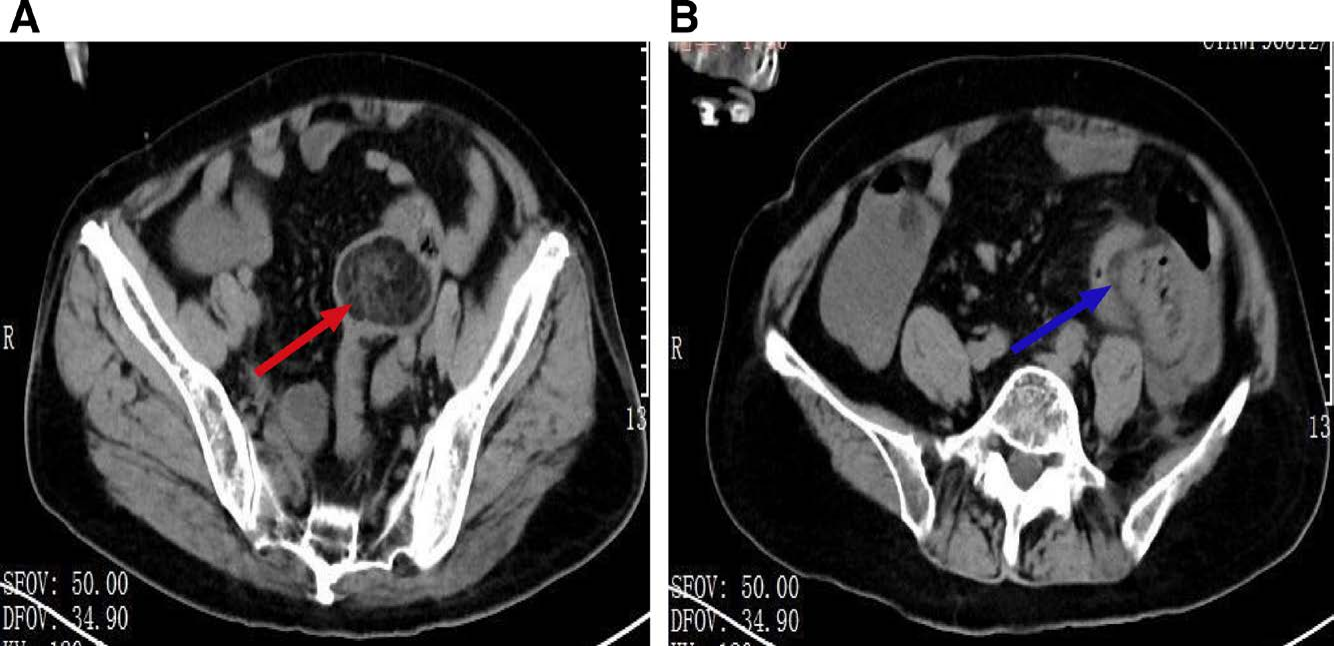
CT examination results. (A) Axial abdominal CT image demonstrates a heterogeneous mass with fatty density within the sigmoid colon (red arrow) and (B) axial abdominal CT image shows intussusception within the abdominal cavity (blue arrow). CT = computed tomography.
